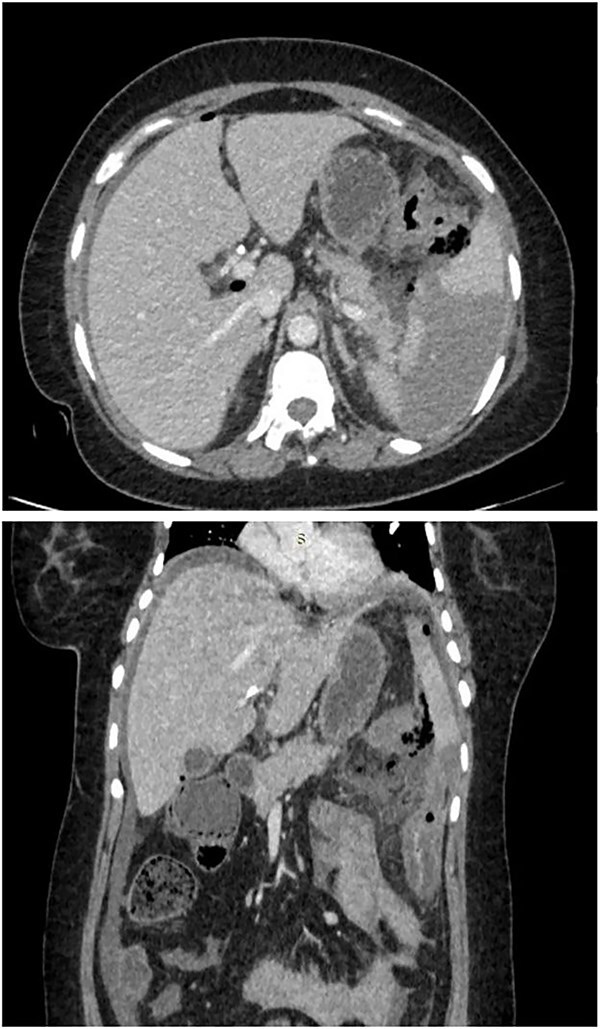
CT scan of abdomen showed pneumoperitoneum in inter bowel space, pancreatic necrosis, peripancreatic collection in splenic flexure region with multiple air foci within it and adjacent mesenteric fat stranding and heterogeneity. (? Splenic flexure of colon perforation).
